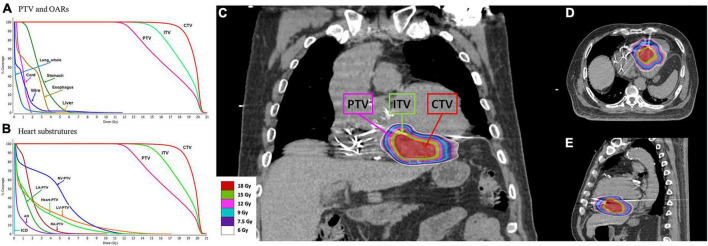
The substrate was targeted at the clinical target volume (CTV). An internal target volume (ITV) was created from the phased-binned 4D CT images to account for the maximum range of motion that was assessed by reviewing the playback of all the phases of the 4D-CT overlaid with the reference CT scan. The planning target volume (PTV) was generated as a 3-mm volumetric expansion from the ITV. The goal was for at least ≥ 95% of the PTV to be covered by at least 95% of the prescribed dose (12 Gy). Optimization was performed to place prescription hotspots (areas receiving > 100% of the prescribed dose) within the ITV and CTV, enabling the ITV and CTV to be covered by up to 125% and 150% of the prescribed dose, respectively. (A) Dose volume histogram (DVH) for the PTV and organs at risk. (B) DVH for the PTV and heart substructures. RV, right ventricle; LV, left ventricle; RA, right atrium; LA, left atrium; AO, aorta; ICD, cardioverter-defibrillator. (C) Coronal view of the plan with color wash. (D) Transverse view of the plan with color wash. (E) Sagittal view of the plan with color wash.
